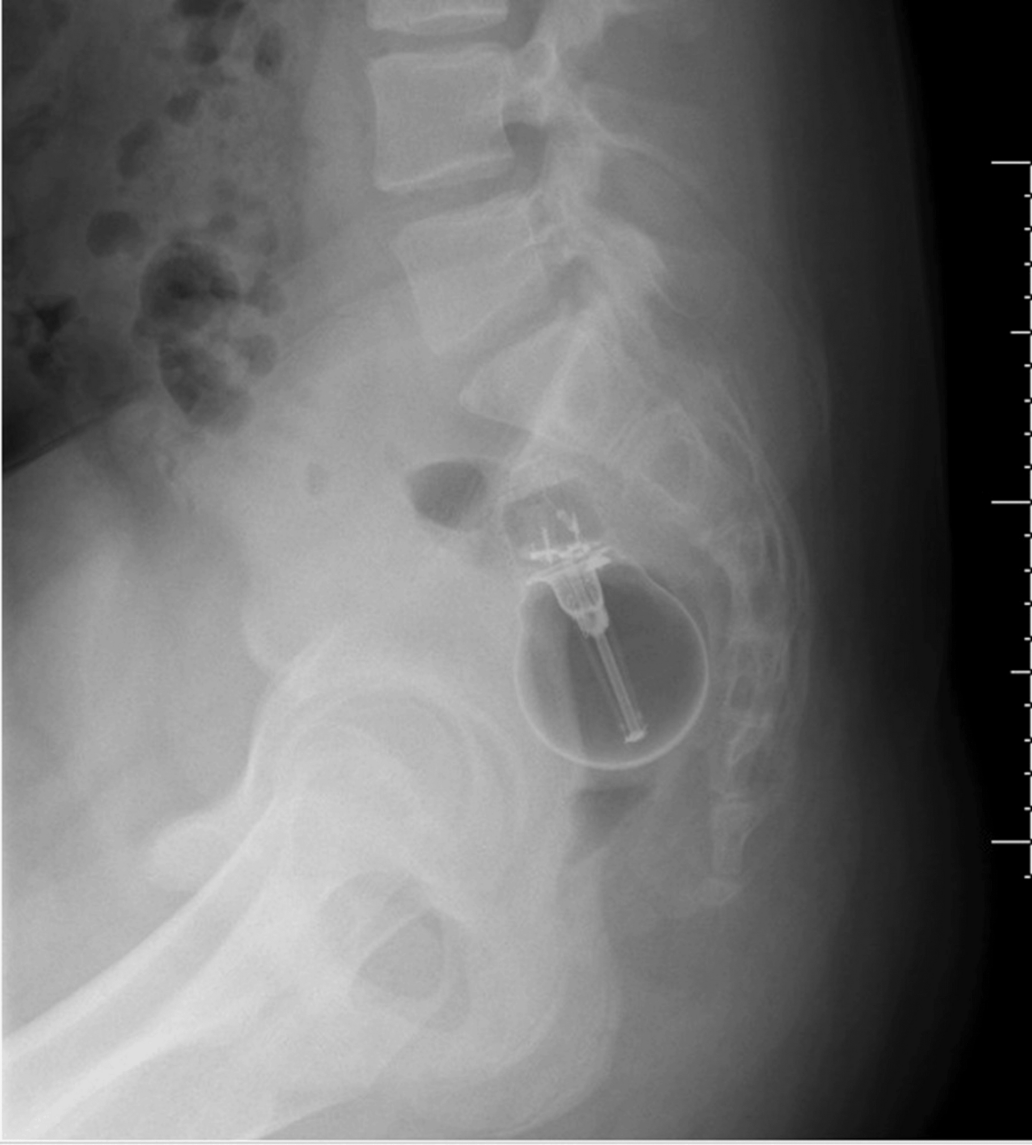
Lateral view X-ray of the pelvis
